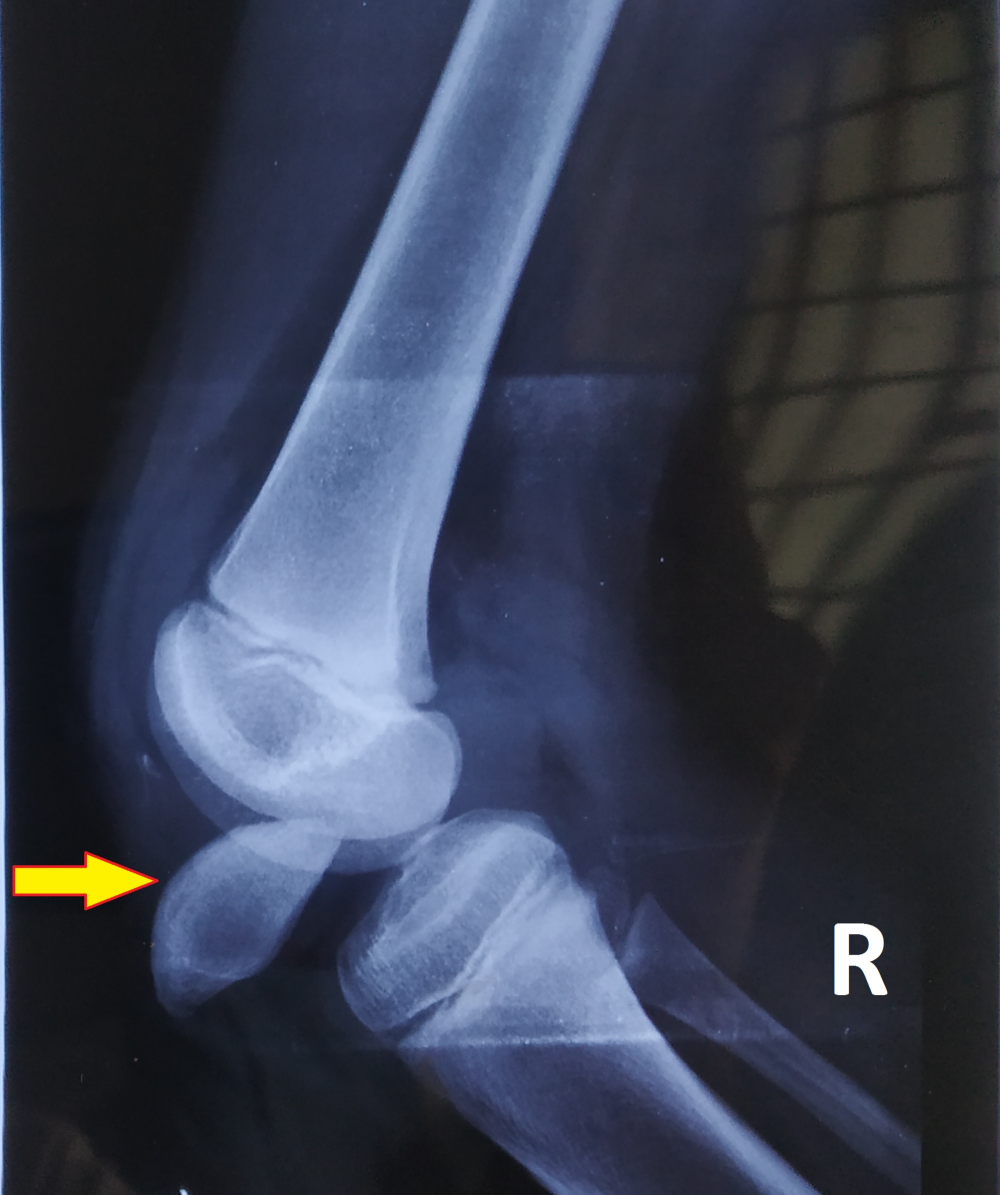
X-ray of the right knee shows intra-articular dislocation of the patella: lateral view
